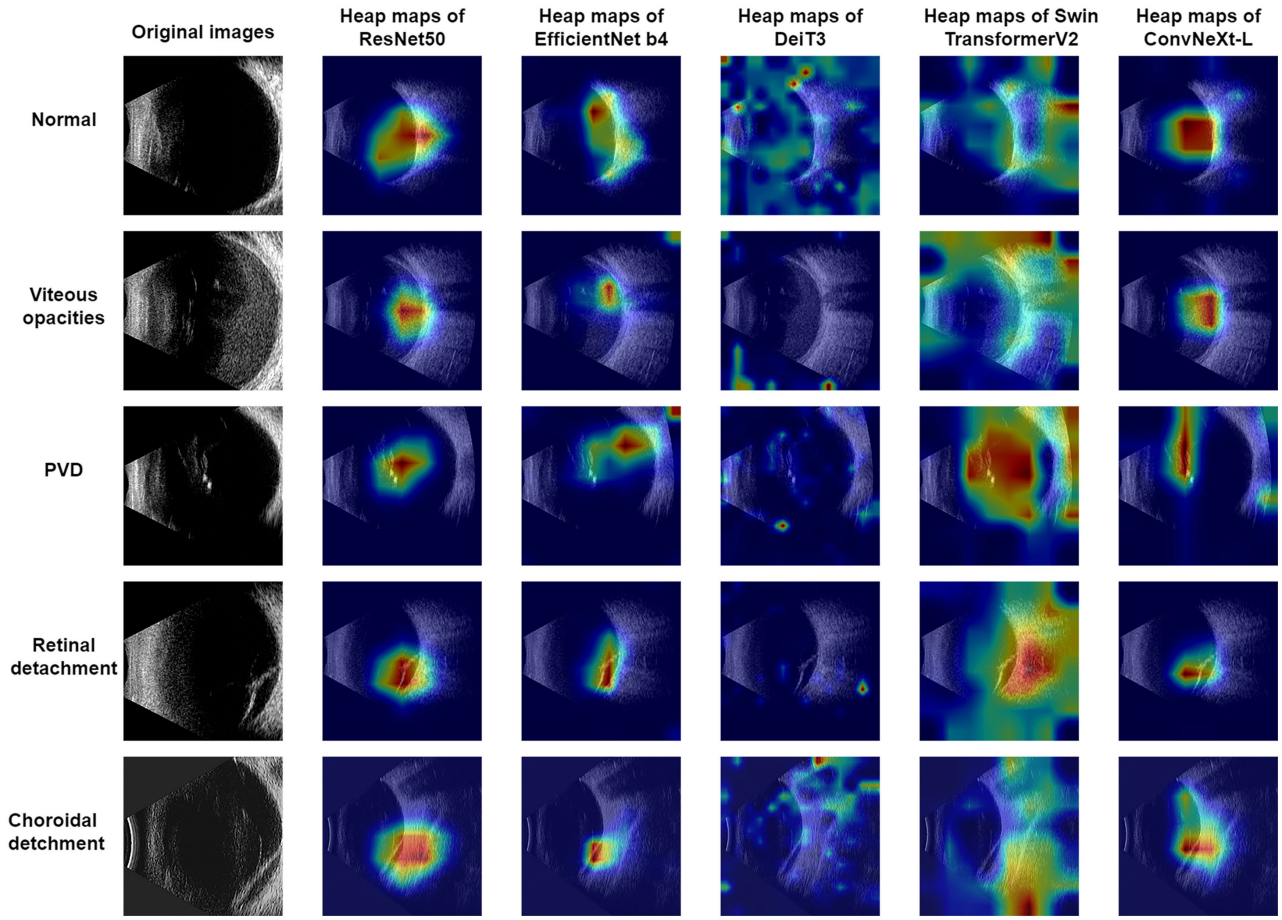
B-mode ultrasound images and four common eye diseases, along with visualization results.
